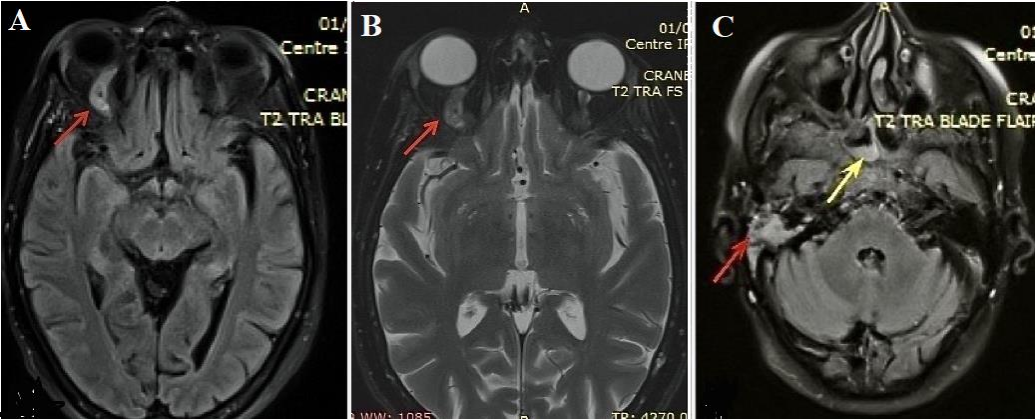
orbito-cerebral angio magnetic resonance imaging; axial images: (A,B) thrombosis of the right superior ophthalmic vein, with inflammatory infiltrate in the upper right rectus muscle; (C) sphenoidal sinusitis, and right otitis media
